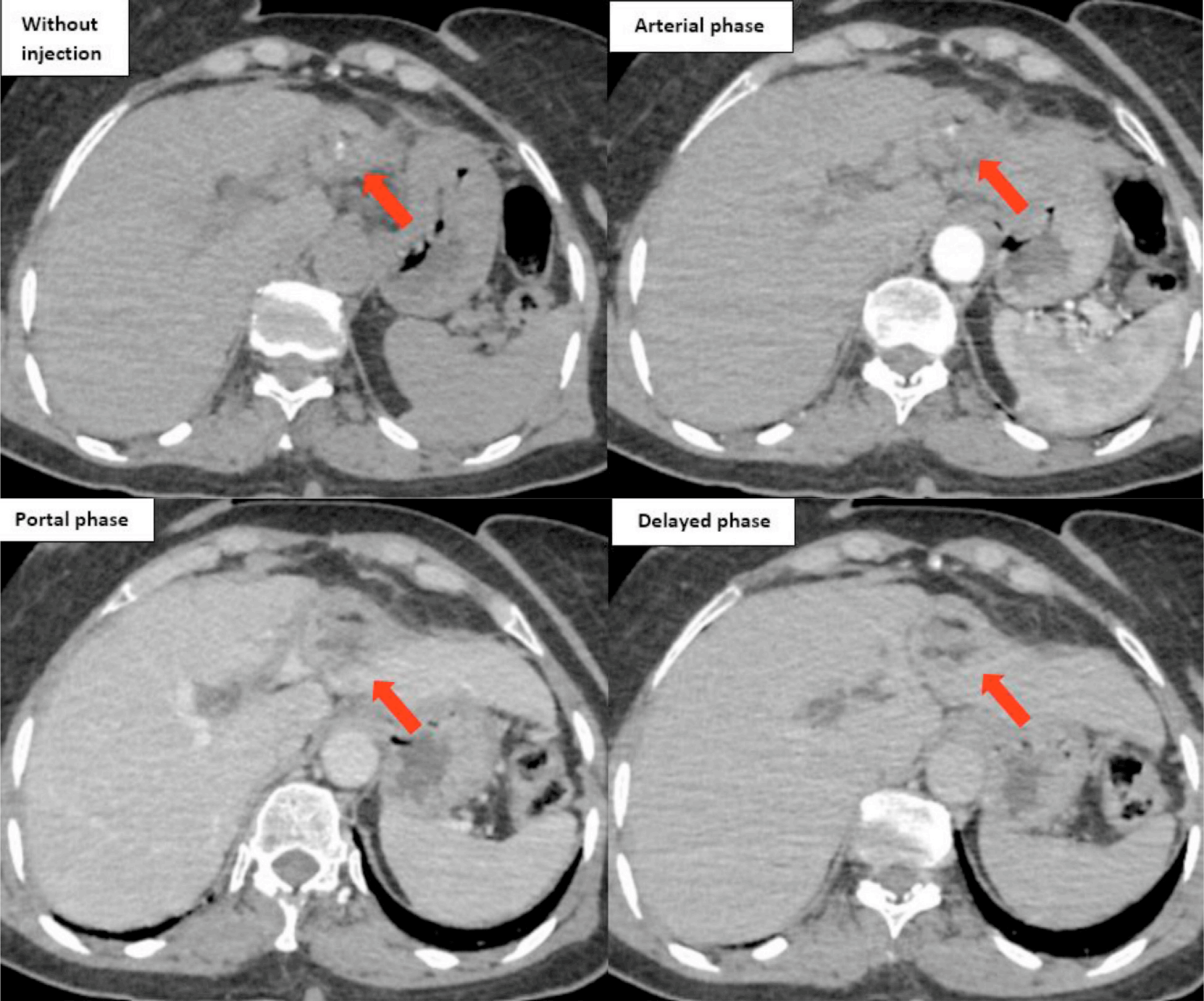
Contrast-enhanced abdominal CT scan (unenhanced and triphasic acquisition). Well-defined, rounded lesion located in hepatic segment IV, appearing hypodense, containing both fatty components and calcifications, with mild peripheral enhancement observed during the portal venous phase following intravenous iodinated contrast injection (red arrow).
